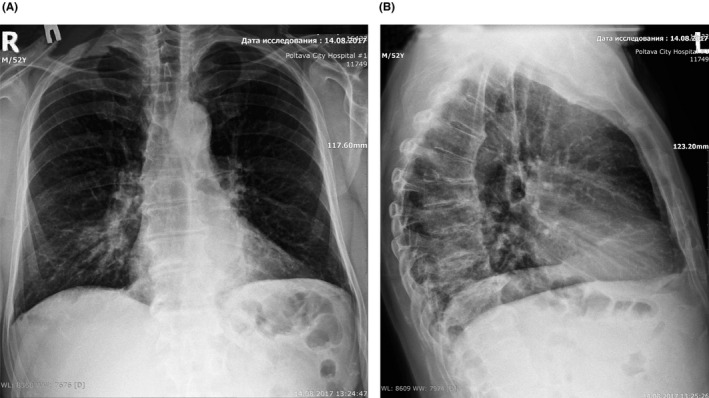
Plane chest radiography of the patient with pneumonia. A, direct projection, B, right side projection
